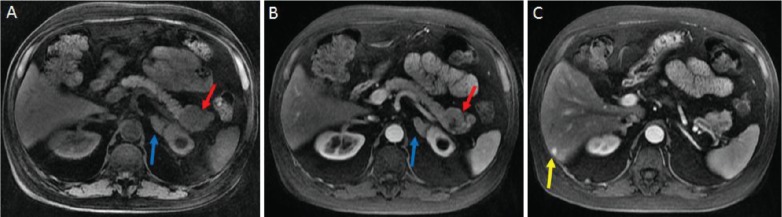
A: magnetic resonance imaging performed in the non-contrasting T1 sequence, showing an enlarged and irregular expansive lesion of the pancreas tail (red arrow) and thickening of the left adrenal (blue arrow). B: magnetic resonance imaging performed in the contrasting T1 sequence. C: nodular lesion in segment VI of the liver (yellow arrow).
